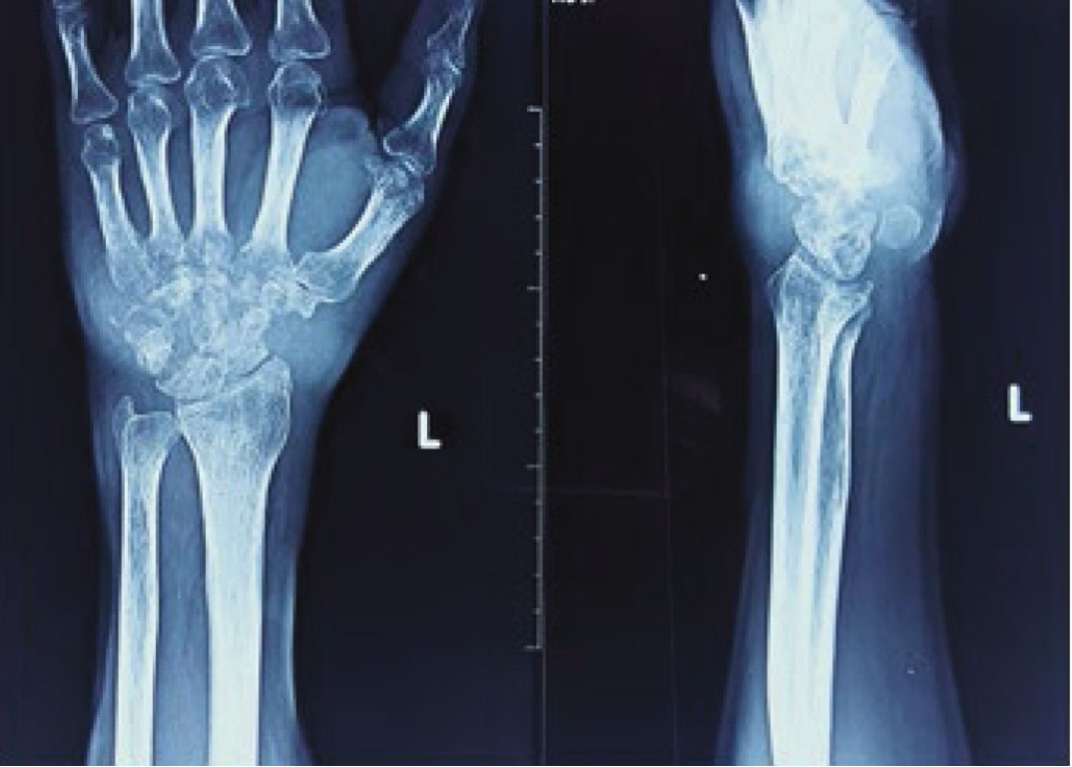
X-ray of the left hand showing destructive type lytic lesions involving mainly the carpal bones and bases of the 2^nd^ to 5^th^ metacarpals.
